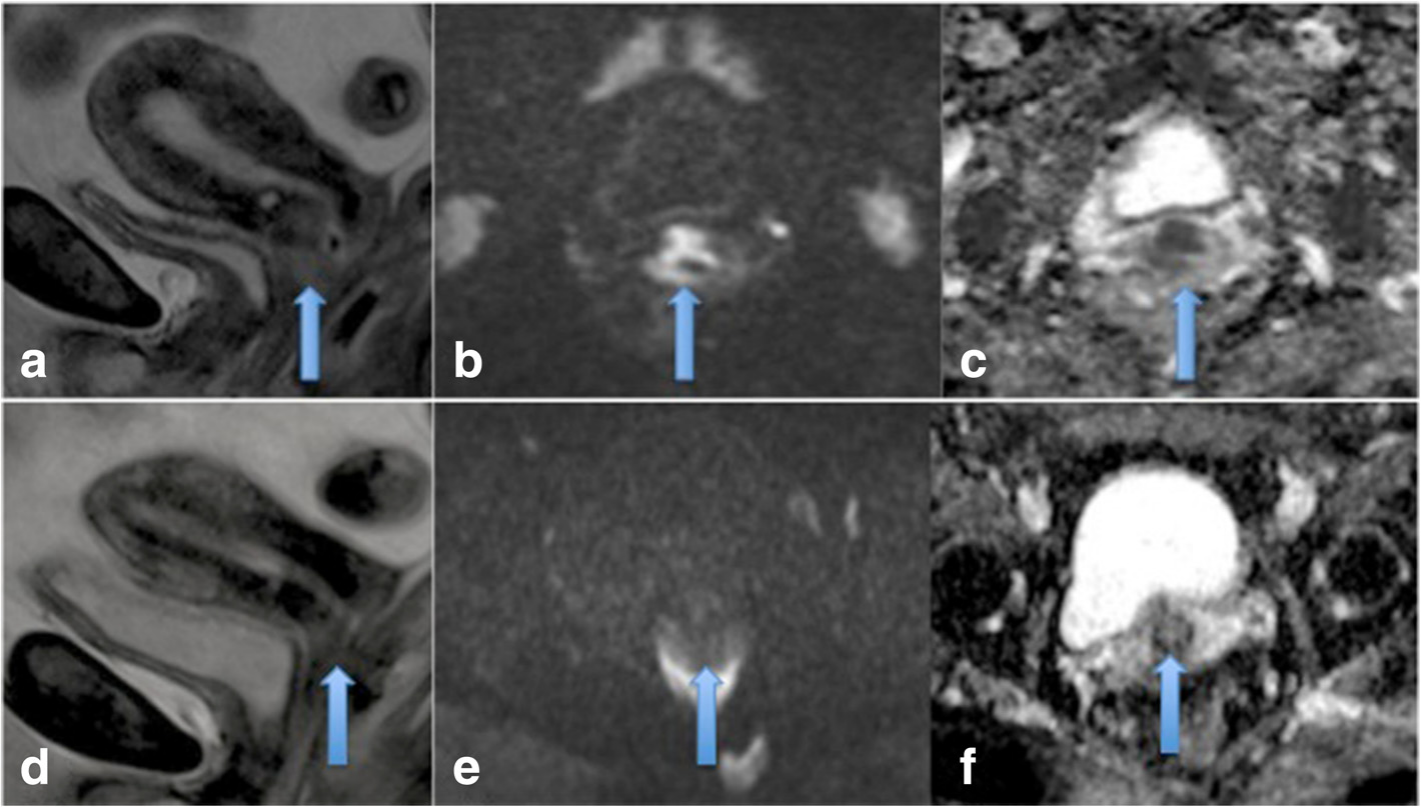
Residual or recurrent disease and post treatment complete response. Sagittal T2 image demonstrating an intermediate T2 signal intensity mass in the cervix which is either residual or recurrent disease (a), axial DWI images through the tumour showing restricted diffusion, high signal on b1200 image (b) and low signal on the corresponding ADC map (c). Post-treatment images (d-f) demonstrating no residual disease on T2 weighted sagittal images and axial DWI images.
